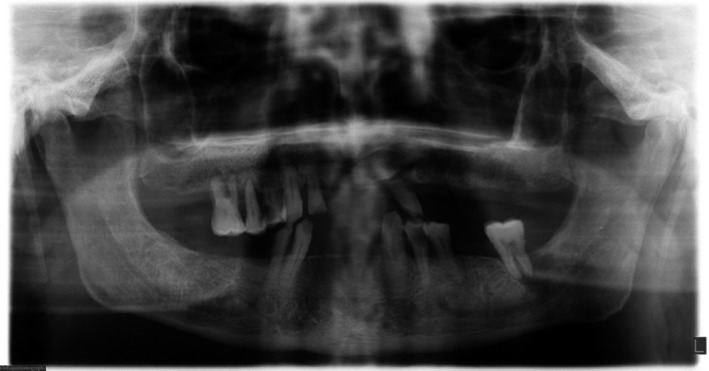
Diagnostic Panoramic Radiography Shows Border Irregularity and Radiolucency in Apical Area of a Second Left Molar in the Mandible
